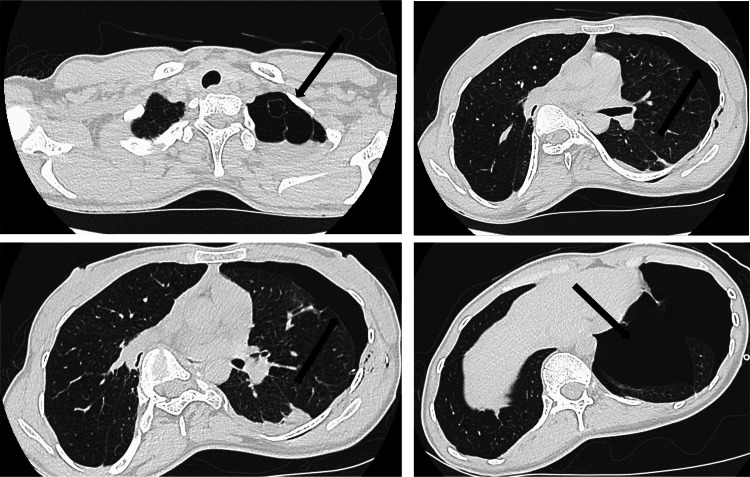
Chest computed tomography findings The chest computed tomography shows the collapse of the left lung and bilateral emphysematous changes.
